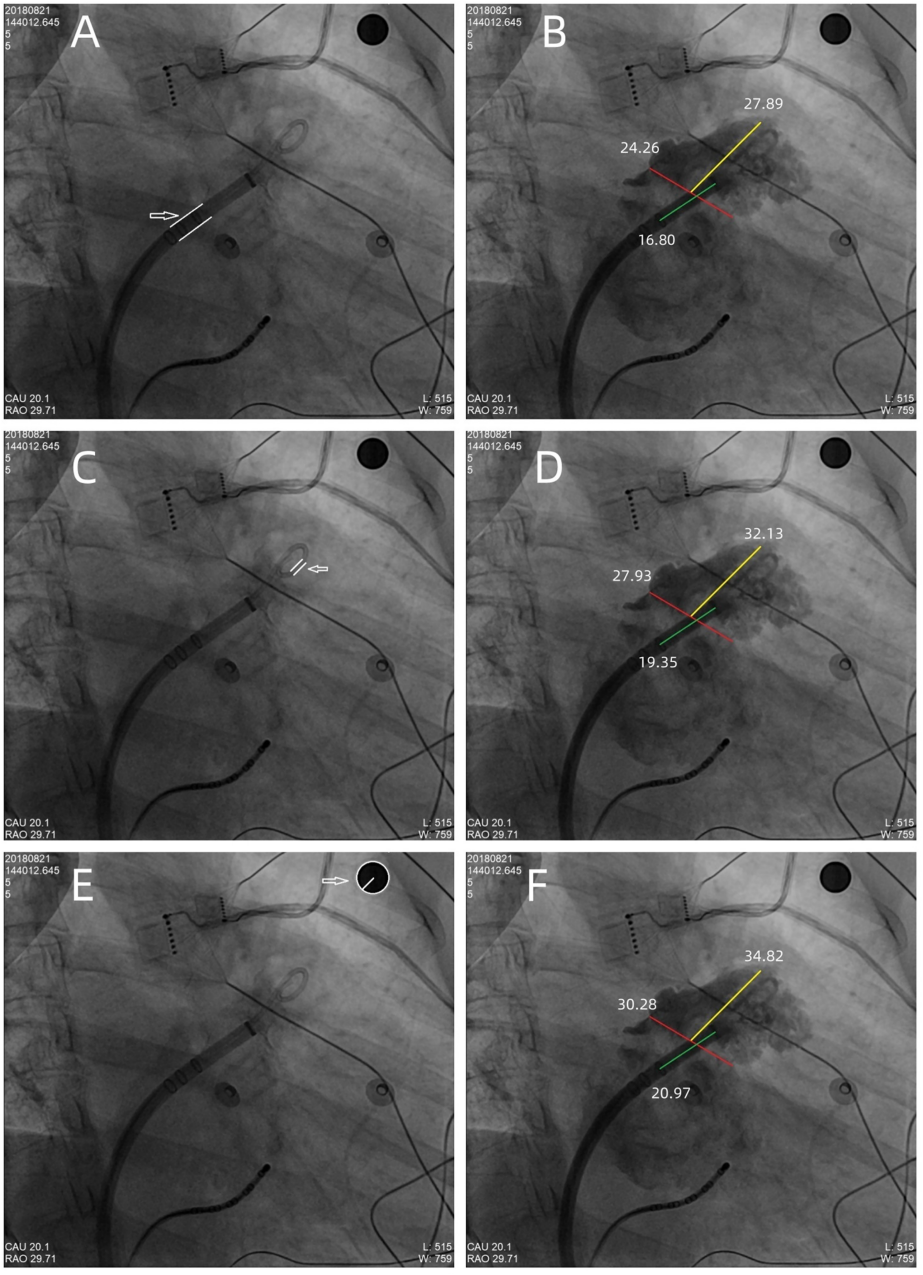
The maximum LAA ostium diameter, maximum LAA depth, and 1st sheath marker band length were measured under x-ray fluoroscopy, using the delivery sheath, pigtail sheath, and steel ball as references, respectively. (A,B) The double white lines pointed by the white arrow showed the 12Fr inner diameter delivery sheath (4 mm in diameter) as reference scale, the red line showed the measurement of maximum LAA ostium diameter (24.26 mm), the yellow line showed the measurement of maximum LAA depth (27.89 mm), and the green line showed the measurement of 1st sheath marker band length (16.80 mm). Similarly, in (C,D), the double white lines showed the 5Fr delivery sheath (1.67 mm in diameter) as reference scale, the red line, yellow line, and the green line showed the measurement of maximum LAA ostium diameter (27.93 mm), maximum LAA depth (32.13 mm), and 1st sheath marker band length (19.35 mm), respectively. (E,F) The white arrow showed the steel ball (10 mm in diameter) as reference scale, the red line, yellow line, and green line showed the measurement of maximum LAA ostium diameter (30.28 mm), maximum LAA depth (34.82 mm), and 1st sheath marker band length (20.97 mm), respectively. In this case, a 33 mm Watchman device was successfully implanted with satisfactory results.
